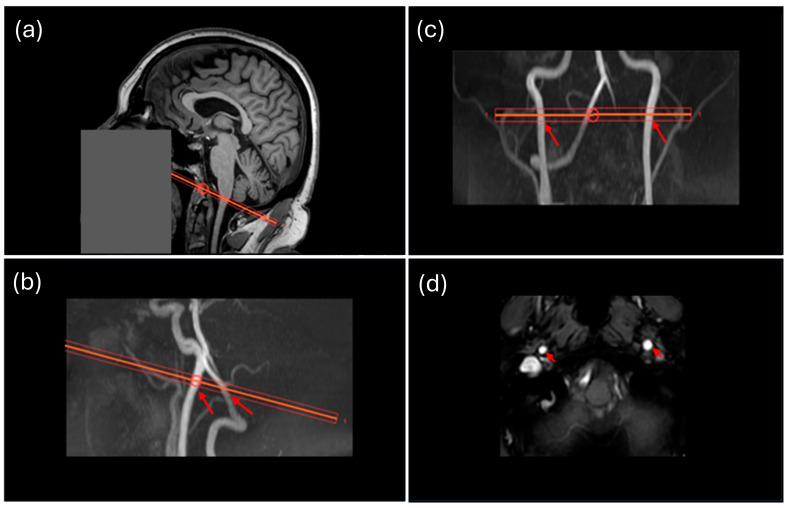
Example MRI acquisition and processing for flow quantification. (a) Sagittal view showing the oblique plane of the imaging placement across the ICAs (orange line, with a circle showing the central point), overlaying on the T1-weighted anatomical image. (b) Sagittal view showing the oblique plane placement of the imaging placement (orange line) crossing the bilateral ICAs (red arrows). (c) Coronal view showing the imaging placement (orange line) crossing the bilateral ICAs (red arrows). (d) Axial view highlighting the bilateral ICAs. Red arrows indicate the bilateral ICAs. Regions of interest (ROIs) of the two ICAs were drawn on this plane for flow velocity calculation within the ICAs.
